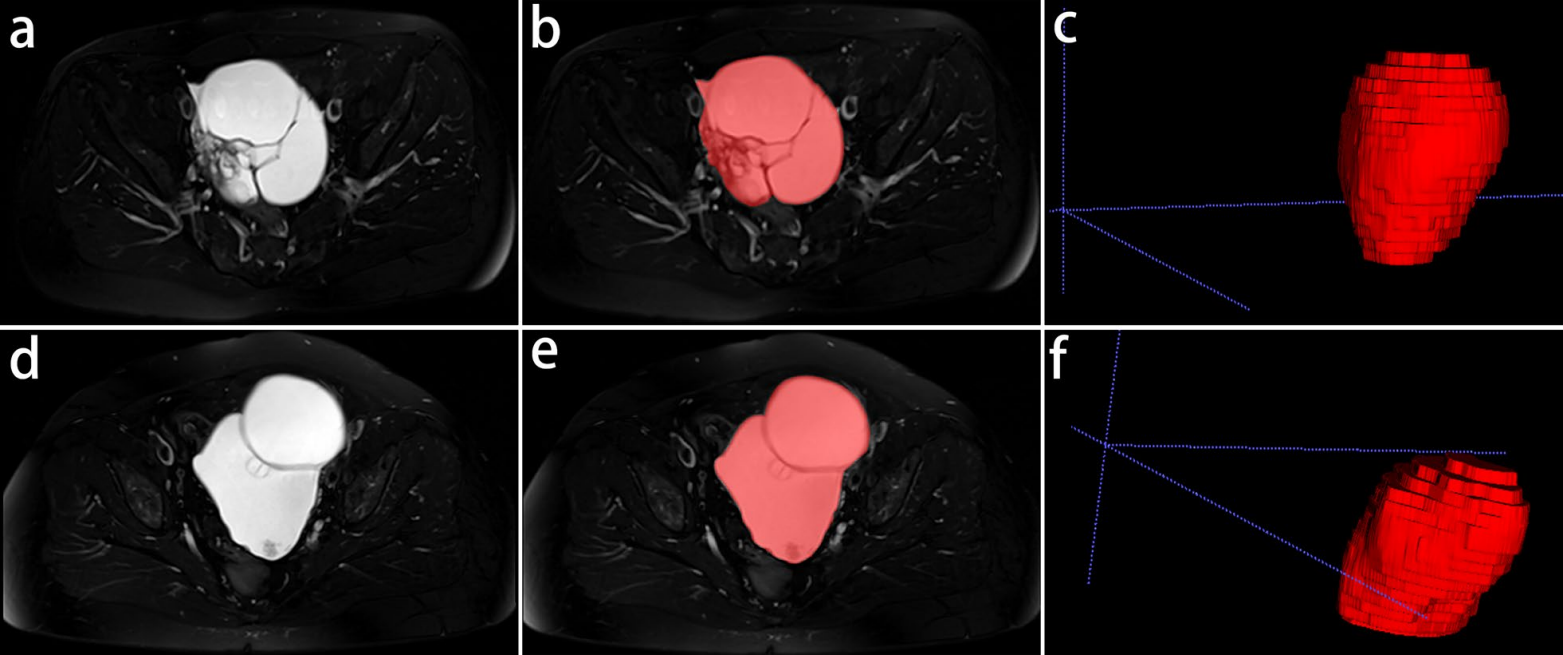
Two representative examples of volumes of interest (VOIs) segmentation of benign and borderline EOTs in axial fat-suppressed T2-weighted images. a–c A 50-year-old woman with a right benign mucinous cystadenoma; d–f a 60-year-old woman with a left borderline mucinous cystadenoma; a, d the original images; b, e the VOIs of ovarian masses showing in red; c, f 3D renderings of the VOIs
